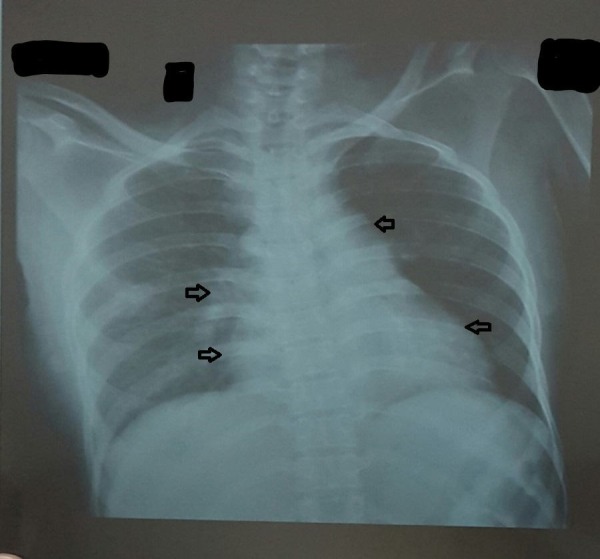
Chest x-ray Arrows pointing towards cardiomegaly.
